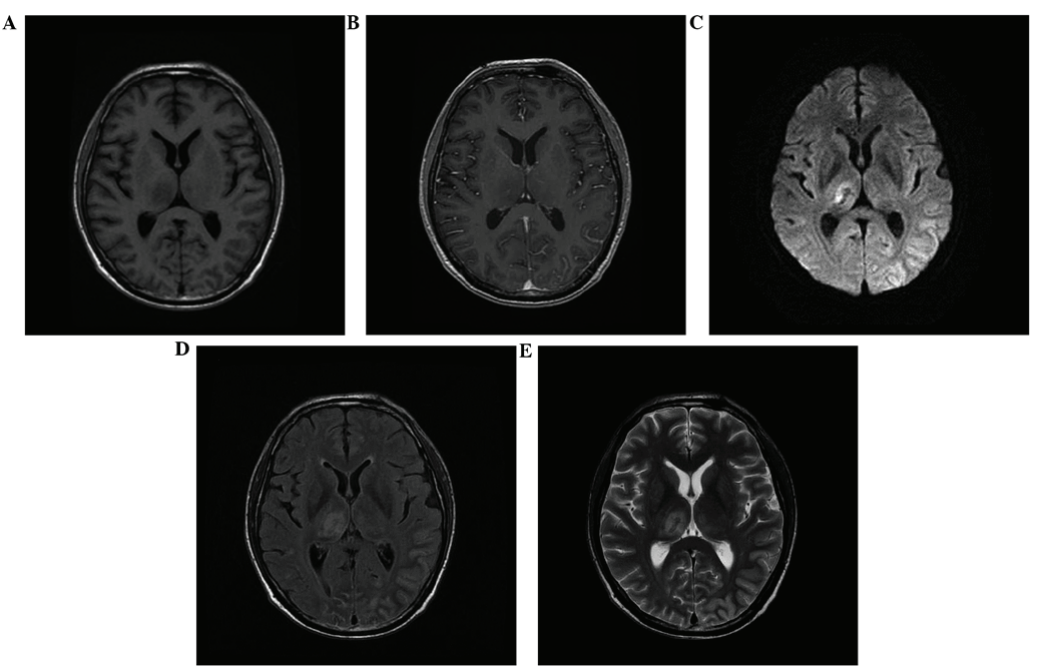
Magnetic resonance imaging scans show cranial nerve nuclei involvement of lymphomatic metastasis. The bilateral cerebral hemisphere cortex had (A and B) marginally long T1 and (C) long T2 signal changes, and cerebral fissure was not apparent. Bilateral basal ganglia had hyperintense T1 and T2 signals. (D) Fluid attenuated inversion recovery showed enhanced signal, whereas (E) diffusion weighted imaging exhibited a slightly low signal on the right. No apparent enhancement of the signal was observed following the injection of the contrast agent.
